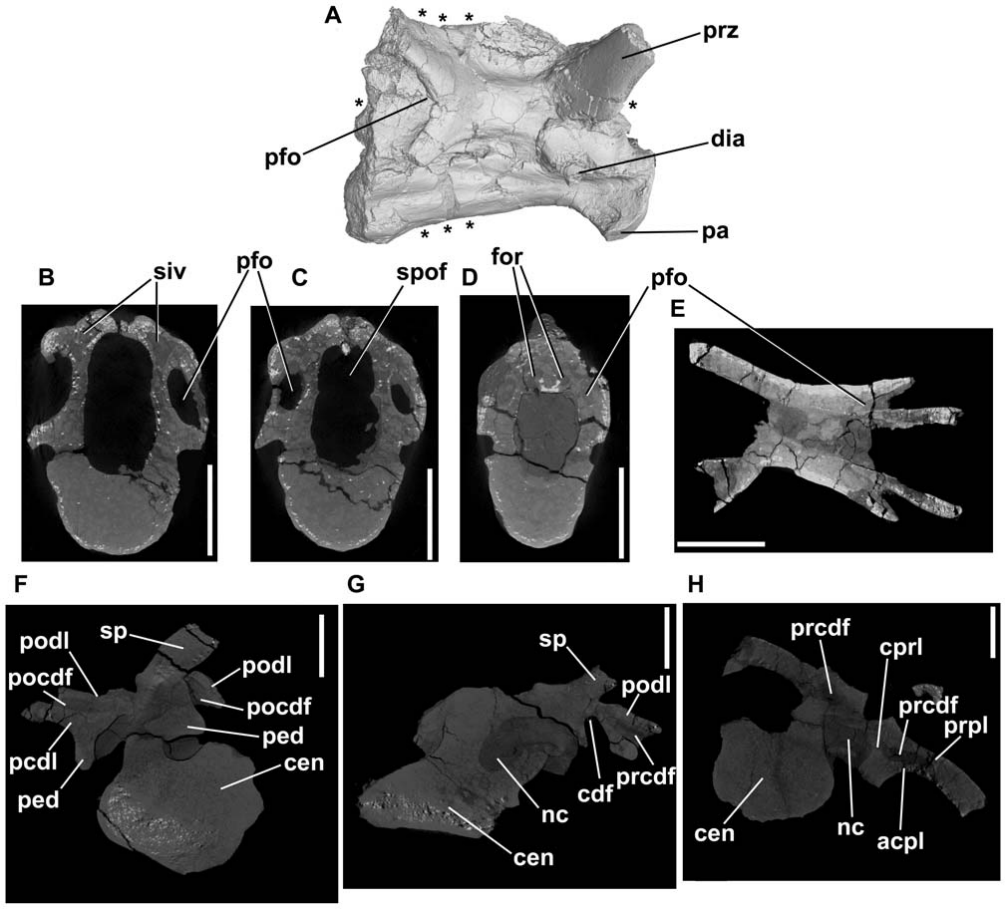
Effigia okeeffeae*, cervical and dorsal vertebrae (AMNH FR 30587). A–E, anterior cervical vertebra in right lateral view (A) and cross-section (B–E). Asterisks dorsal and ventral to the vertebra in A indicate the positions of the transverse sections shown in B–D. Asterisks to left and right of the vertebra in A indicate the position of the axial section shown in E. F–H: CT slices showing transverse sections through AMNH FR 30587, four semi-articulated dorsal vertebrae. F: section through third preserved dorsal, immediately posterior to transverse process. G: section through third preserved dorsal, immediately posterior to transverse process. H: section through second preserved dorsal, immediately anterior to most anterior extent of neural spine. Note that in both vertebrae figured the neural arch and centrum are disarticulated. Abbreviations: acpl, anterior centroparapophyseal lamina; cdf, centrodiapophyseal fossa; cen, centrum; cprl, centroprezygapophyseal lamina; dia, diapophysis; for, foramina within base of spinopostzygapophyseal fossa; nc, neural canal; pa, parapophysis; pcdl, posterior centrodiapophyseal lamina; ped, neural arch peduncle; pfo, deep fossa on posterior of neural arch; pocdf, postzygapophyseal centrodiapophyseal fossa; podl, postzygodiapophyseal lamina; prcdf, prezygapophyseal centrodiapophyseal fossa; prpl, prezygoparapophyseal lamina; prz, prezygopophysis; siv, sediment-infilled vacuity within neural arch; sp, neural spine; spof, spinopostzygapophyseal fossa. All scale bars equal 10 mm.
